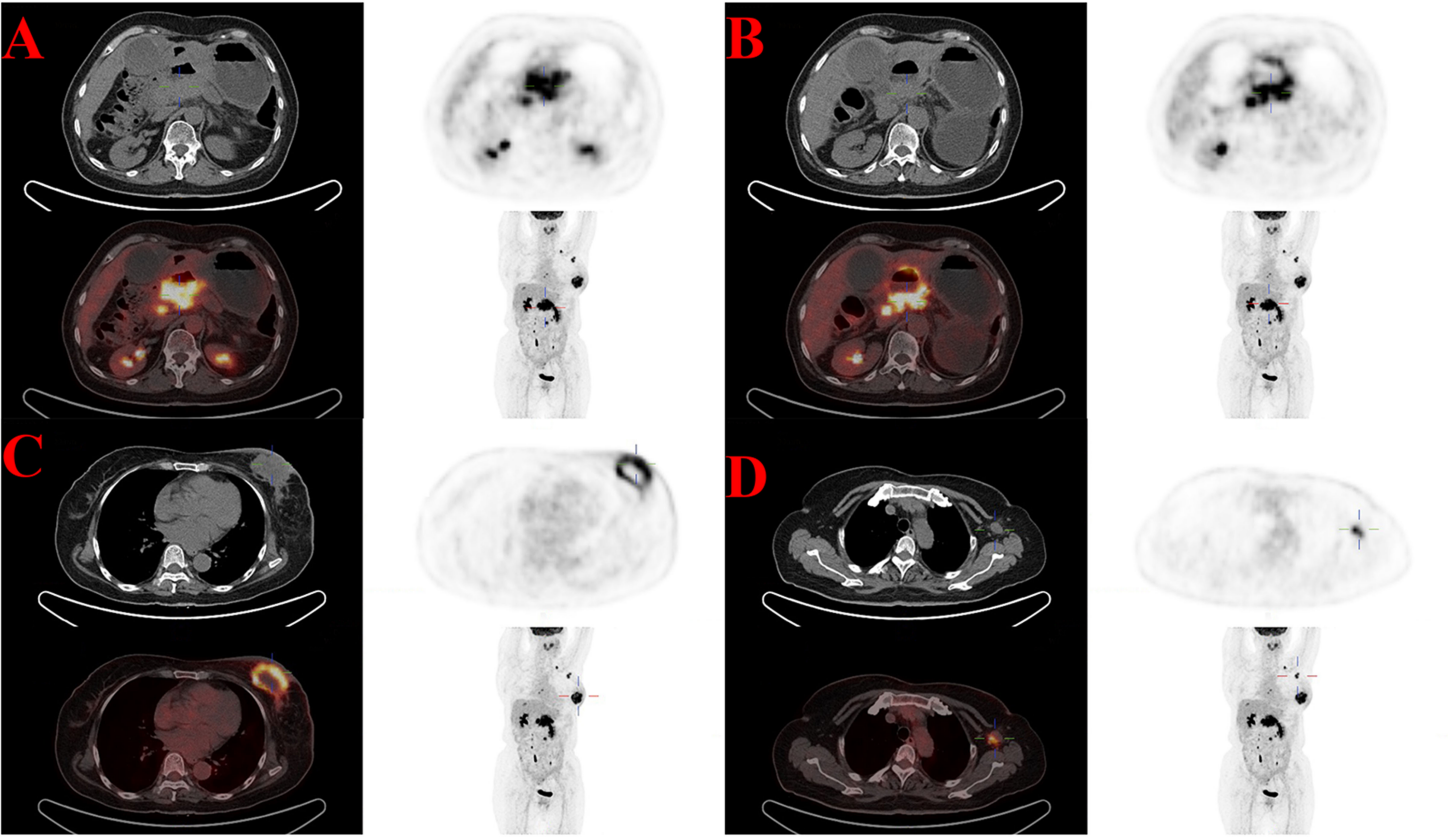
High uptake of 18F-FDG in the known lesions with increasing radioactivity uptake of 18F-FDG PET/CT in September 2022. (A) The hepatopancreatic lesions (SUVmax = 19.8). (B) The dilatation of the distal main pancreatic duct and the intrahepatic bile duct system. (C) The breast lesion (SUVmax = 12.9). (D) Multiple axillary lymph nodes lesions (SUVmax = 10.4).
